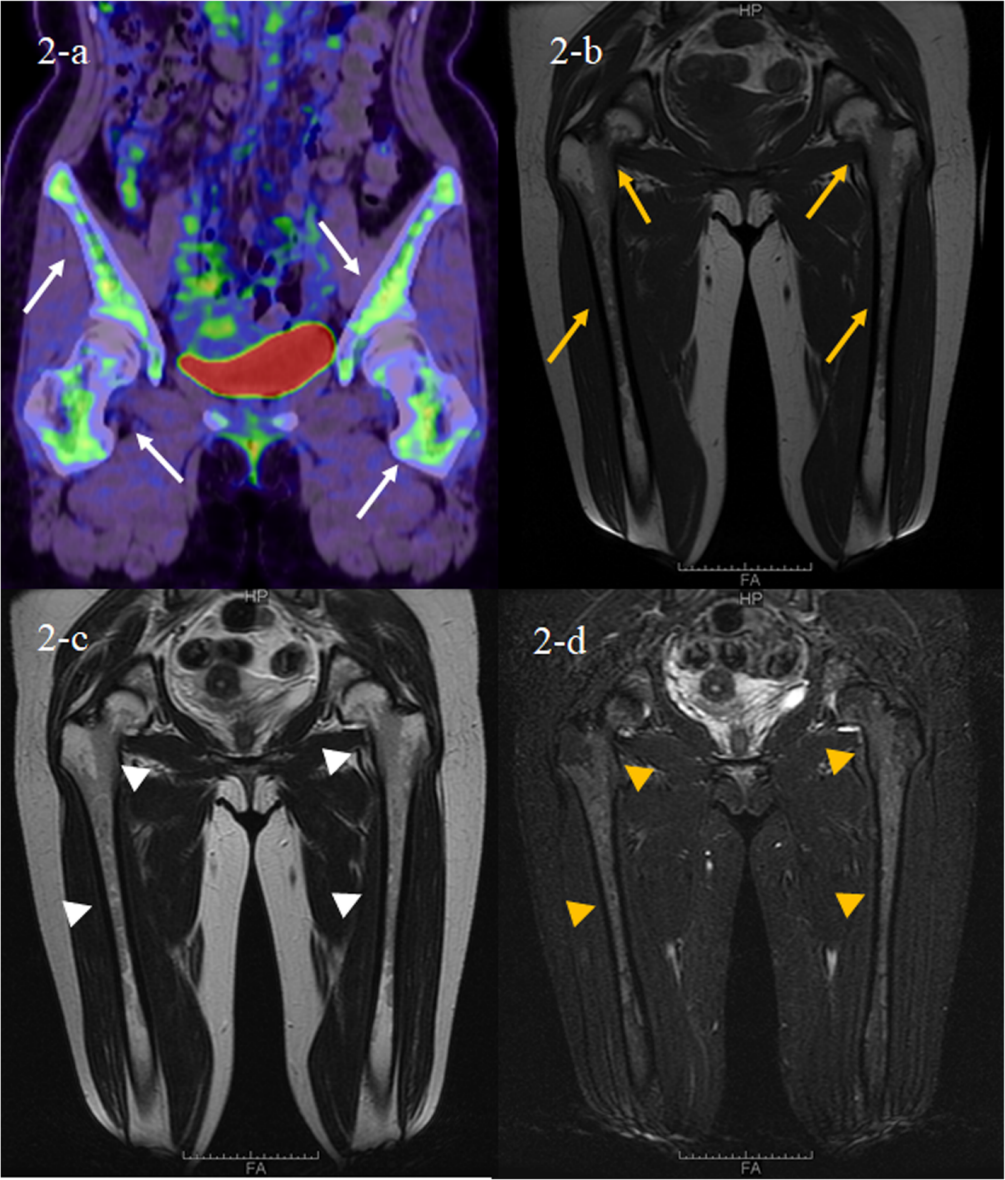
FDG-PET/CT and MRI findings. The FDC-PET/CT findings of femurs demonstrate increased ^18^FDG uptake in the bone marrow at the pelvis and femurs (a, white arrows). The MRI findings of femurs demonstrates low signal intensity on the T1-weighted image (b, yellow arrows) and T2-weighte image (c, white arrowheads) whereas high signal intensity on the STIR image (d, yellow arrowheads). ^18^FDG = fluorine-18-fluro-deoxyglucose, PET/CT = positron emission tomography/computed tomography, MRI = magnetic resonance imaging, STIR = short T1 inversion recovery
